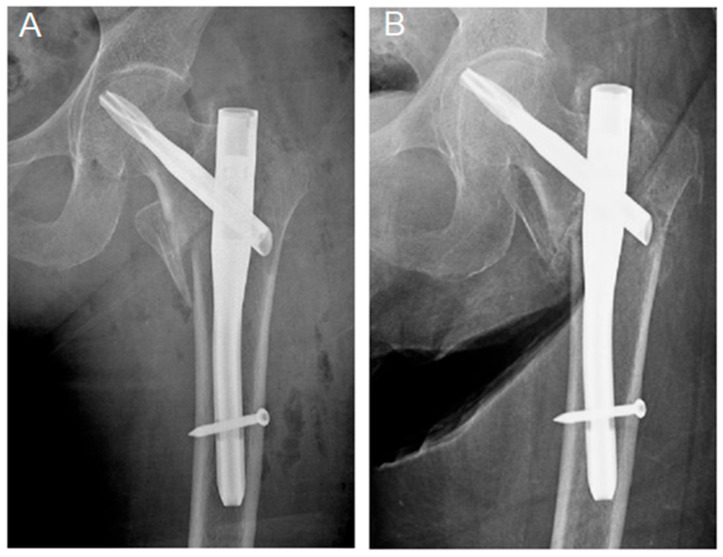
(A) Immediate post. (B) 3-month postoperative radiographs of an 81-year-old female with intertrochanteric fractures with improper proximal fixation (lag device not engaging an incompetent lateral femoral cortex) of a short cephalomedullary nail. The last radiograph (B) shows varus fracture displacement and lag screw articular penetration. The patient declined further interventions and expired 5 months later due to heart disease.
